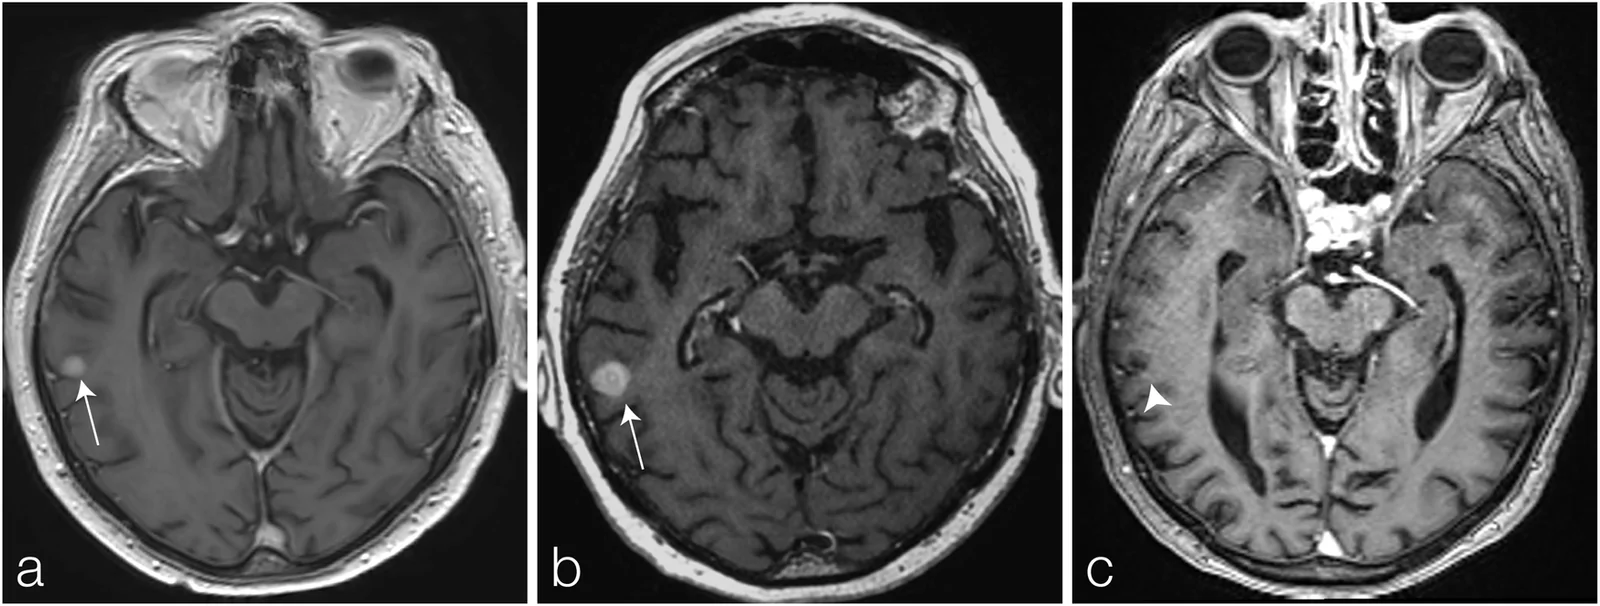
Brain metastasis treated with stereotactic radiosurgery (SRS) and immune checkpoint inhibitor therapy. a Pretreatment axial post-contrast T1-weighted image (arrow). b Follow-up at 4 months demonstrating transient enlargement of the treated lesion (arrow). c Follow-up at 8 months showing reduction in size (arrowhead). The findings are consistent with pseudoprogression in the setting of combined therapy; SRS-related and immune-related contributions overlap and cannot be reliably distinguished on imaging
